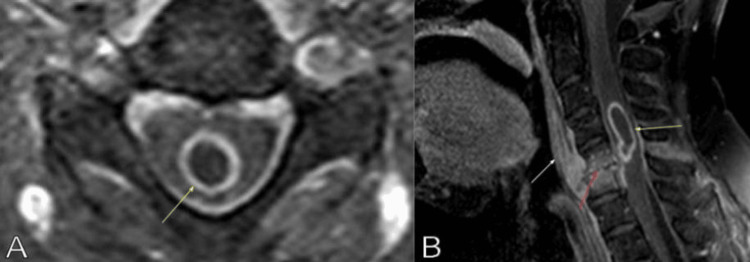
Cervical magnetic resonance imaging T1-weighted with gadolinium. (A) Axial and (B) sagittal images showing the intramedullary abscess (yellow arrow) associated with the C5-C6 discitis and osteomyelitis (red arrow) and the infectious process extending into the prevertebral/retropharyngeal space (white arrow).
